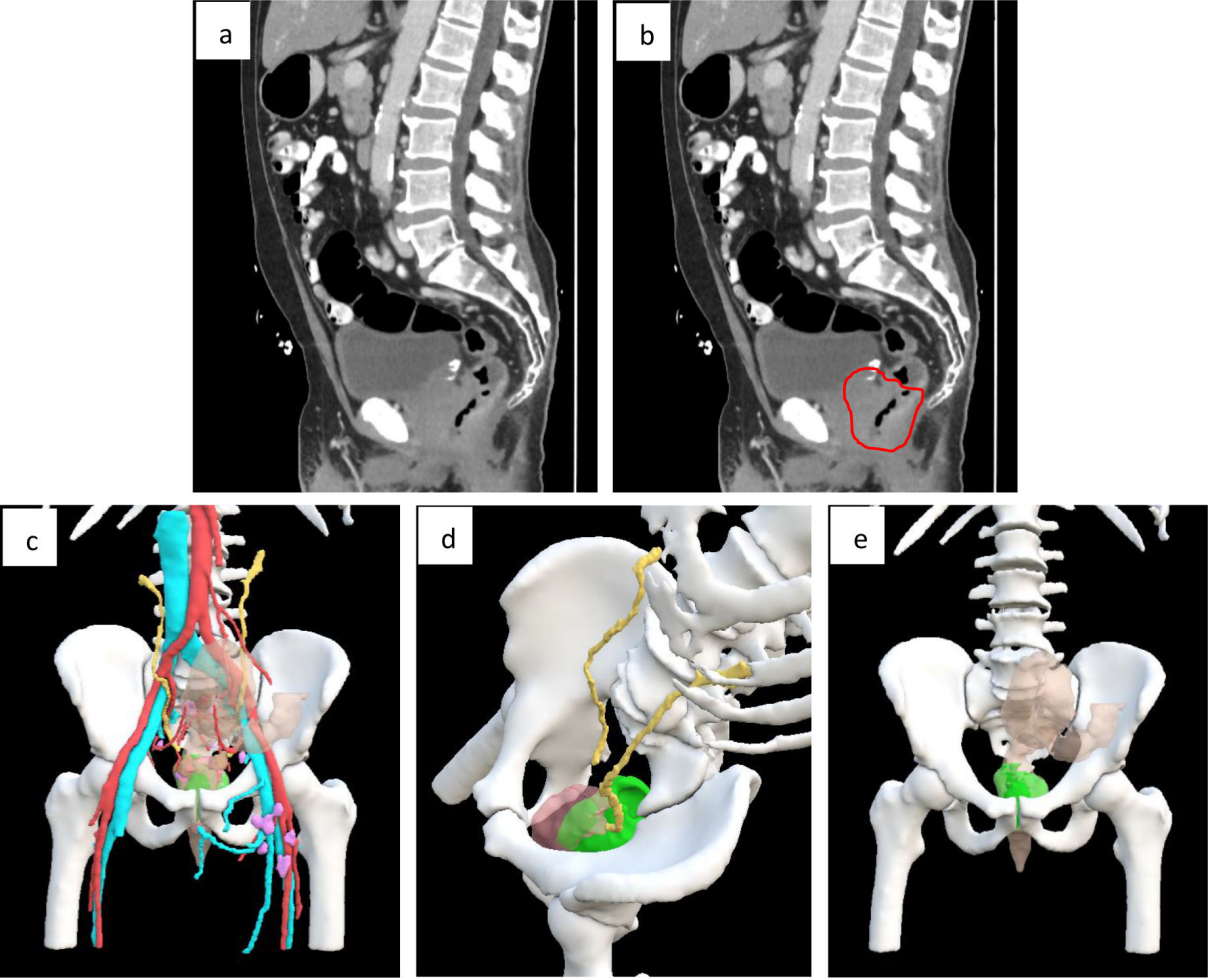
An example of the reconstituted images produced using the software. (a) A sagittal image of a CT scan demonstrating a locally advanced mucinous rectal cancer invading the prostate (b) A sagittal image of a CT scan demonstrating a locally advanced mucinous rectal cancer invading the prostate with tumour highlighted (c) An antero-posterior view of the reconstituted 3D image (d) An oblique view of the reconstituted 3D image (e) An antero-posterior view of the reconstituted image with additional anatomical structures removed to demonstrate the tumour along.
